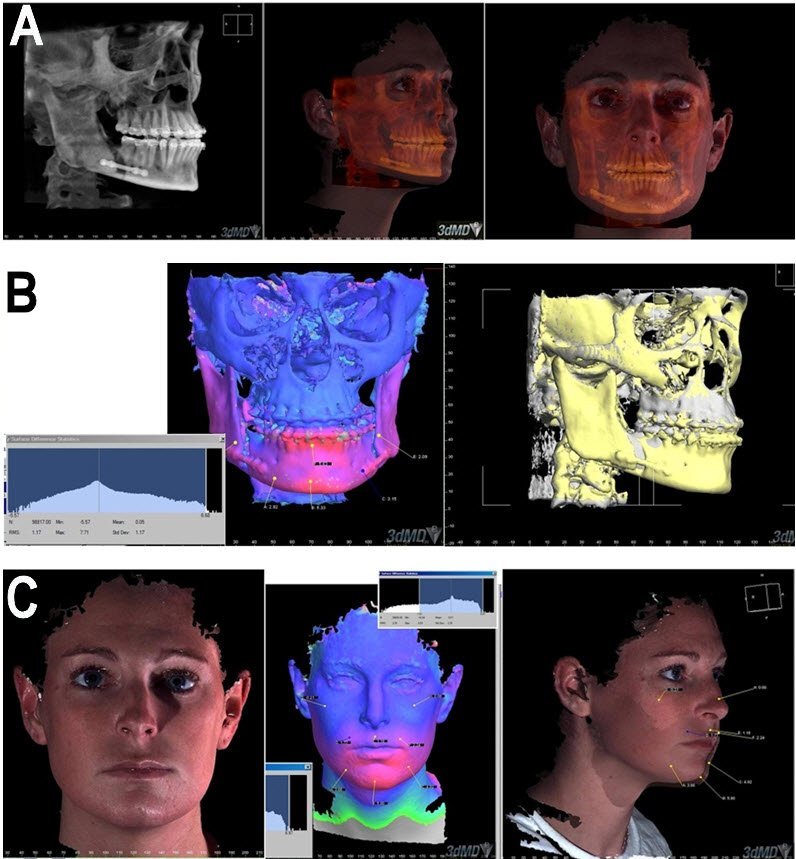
The same patient depicted in Figure 6, post-surgery imaging. A: Patient’s specific anatomical reconstruction with CBCT imaging. B: Actual bone change from treatment. C: Actual soft-tissue change from treatment.
